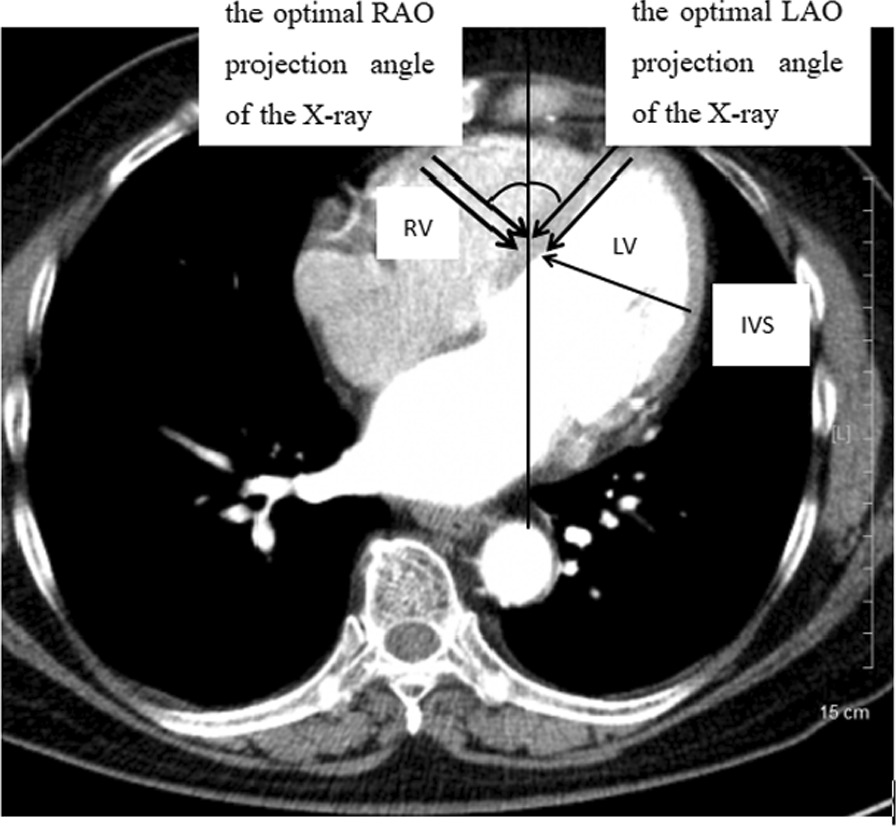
The optimal projection angle of X-ray under cardiac computerized tomography (CT). *RAO* right anterior oblique; *LAO* left anterior oblique; *RV* right ventricle; *LV* left ventricle; *IVS* interventricular septum
